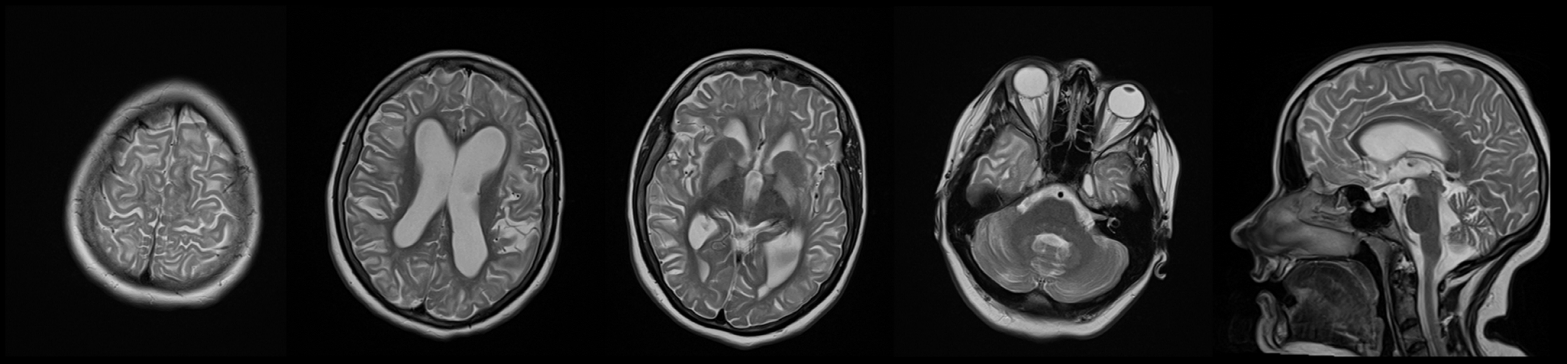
T2-weighted hyperintensity involving the subcortical white matter, globus pallidus, and dentate nucleus of the cerebellum in patient carrying pathogenic variant in *L2HGDH* gene (Case 2).
